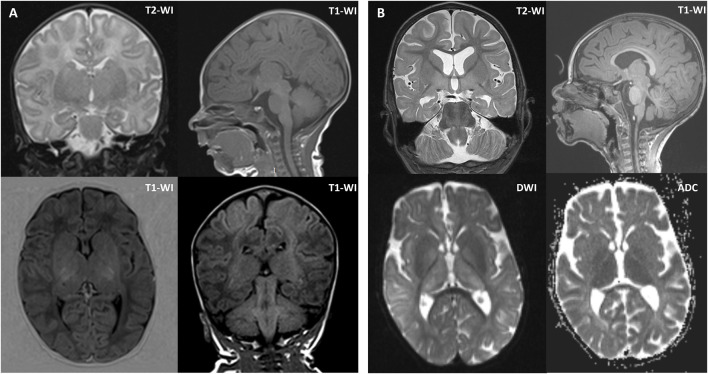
Brain MRI of the proband at 1 month of age (A) and 2 years and 1 month of age (B). (A) Brain MRI at 1 month of age showed symmetrically increased MR signal and volume of the putamen and thalamus. (B) Brain MRI at 2 years and 1 month of age showed mild diffuse cerebral atrophy without cortical architectural disruption, except for hypoplasia of the corpus callosum, periventricular white matter alterations, hypomyelination of the deep white matter, and symmetrical enlargement of the putamen, thalamus, and red nucleus. Axial diffusion-weighted imaging (DWI) and apparent diffusion coefficient (ADC) images showed restricted diffusion in the basal ganglia. Coronal T2-weighted images (T2-WI) at 1 month of age and 2 years and 1 month of age revealed mild progression of cerebral atrophy. Sagittal T1-weighted images (T1-WI) at 1 month of age and 2 years and 1 month of age showed mild progression of hypoplasia of the corpus callosum.
